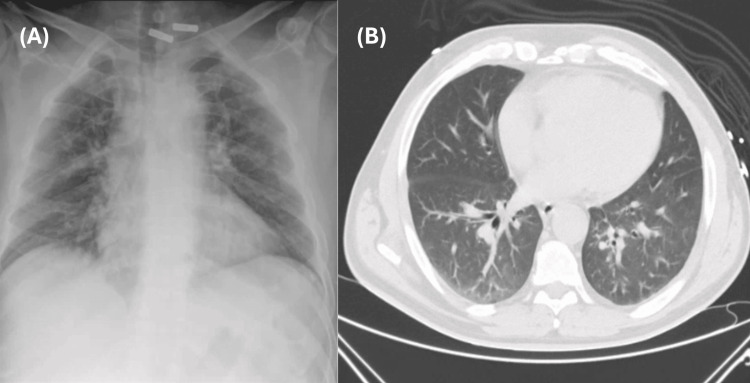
(A) Chest X-ray and (B) thoracic abdominal pelvic CT scan (A) Chest X-ray showing patchy infiltrates. (B) Contrast-enhanced thoraco-abdomino-pelvic CT angiography showing no evidence of pulmonary thromboembolism. The abdominal aorta, celiac trunk, superior mesenteric artery, and renal arteries are patent, with no signs of occlusion and no abnormal abdominal organ findings.
